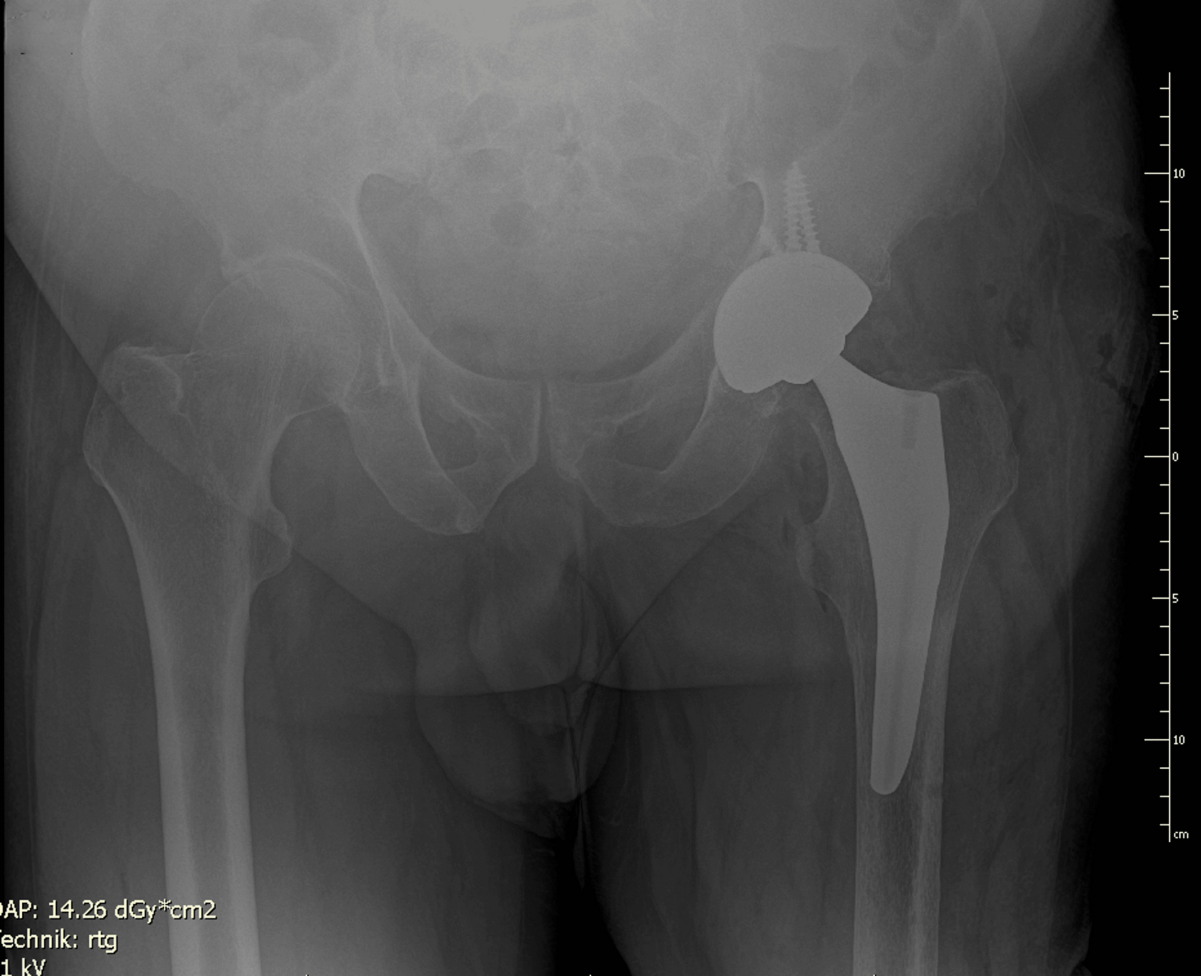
Immediate postoperative AP radiograph showing stable acetabular and femoral components.
